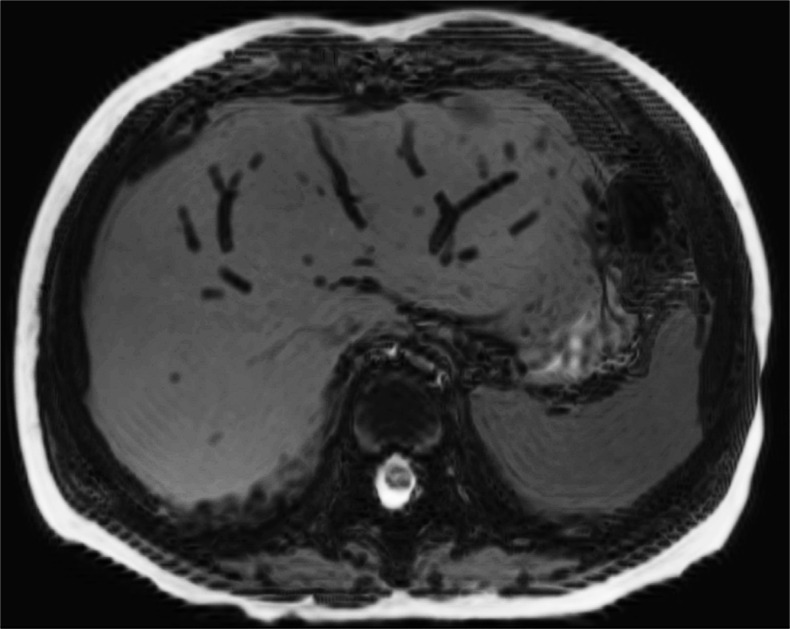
Hepatobiliary gas. Axial minimum-intensity projection T2-weighted postmortem magnetic resonance imaging image following neonatal death shows hepatobiliary air, likely in the hepatic veins in this case
